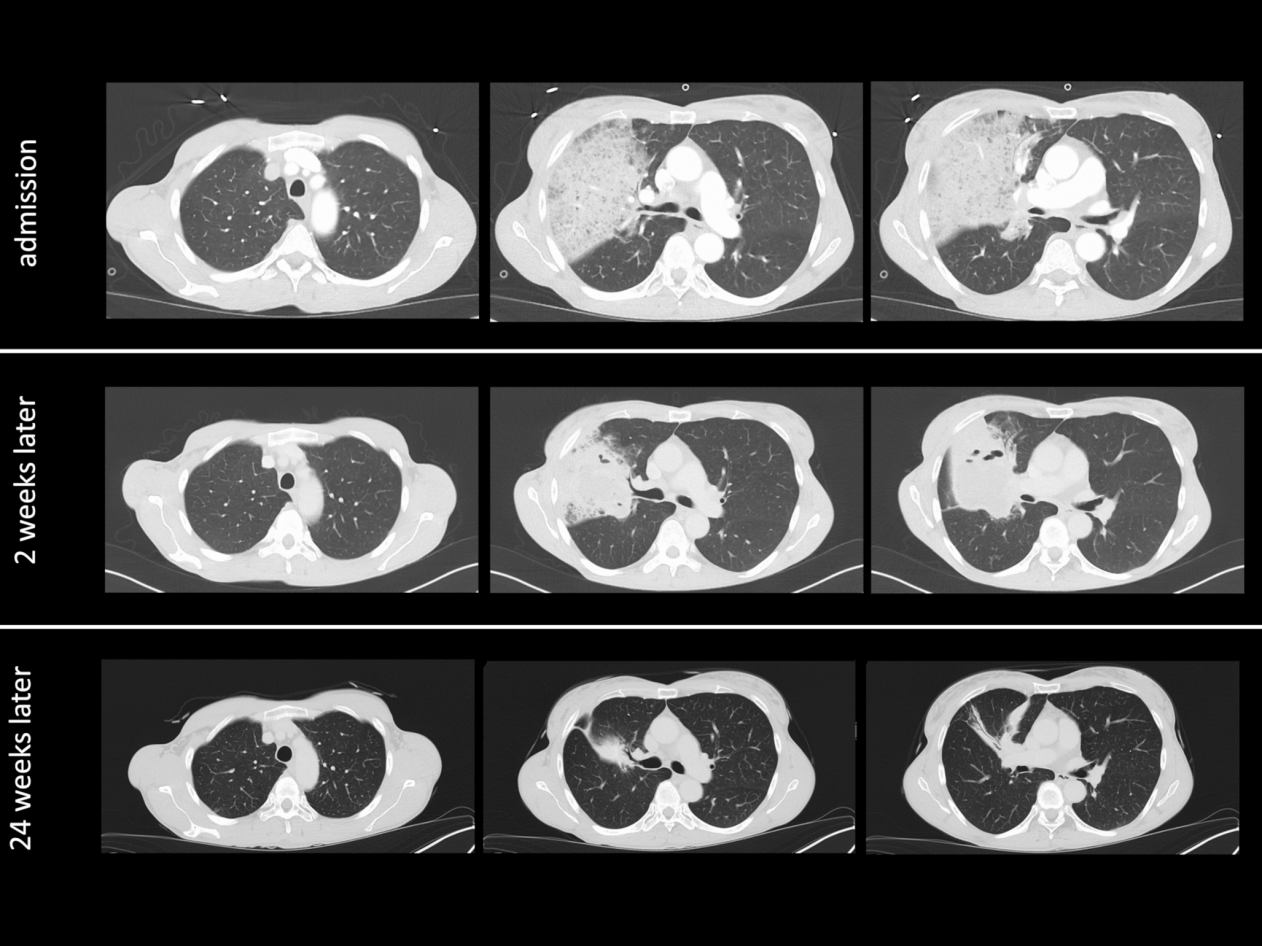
Chest CT scans in the lung window at various time points during the course of the necrotizing lung infection. Chest CT scans of three time points are shown: Time of admission, two weeks after admission when the lung abscess first became apparent, and at 24 weeks after admission when only residual changes were observed and antibiotic therapy was discontinued. For each time point three images are presented: At the levels of the aortic arch, the tracheal bifurcation and the bronchial segmentation
